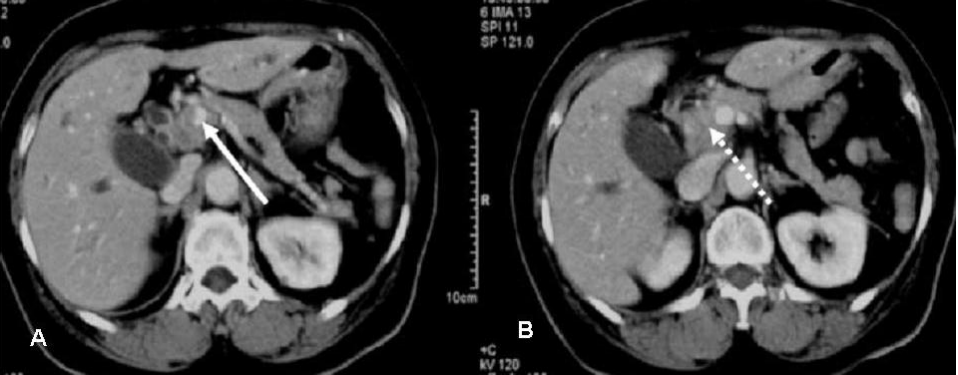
a and b. Computed tomography shows the PVTT (white arrow) and bile duct cancer (white dotted arrow).
